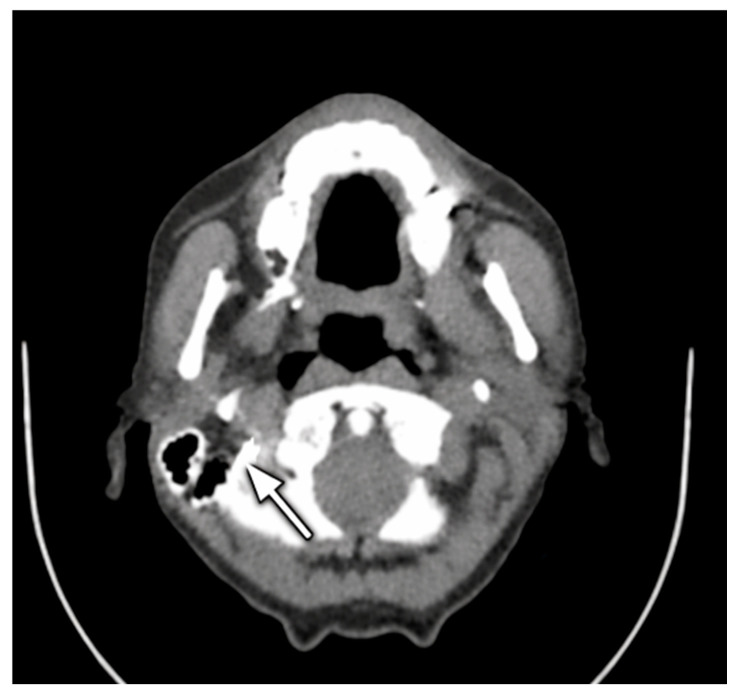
Axial contrast-enhanced CT scan of the neck showing a retroesophageal abscess with intralesional air bubbles. The arrow indicates the suspected cervical esophageal fistulous tract communicating with the collection.
